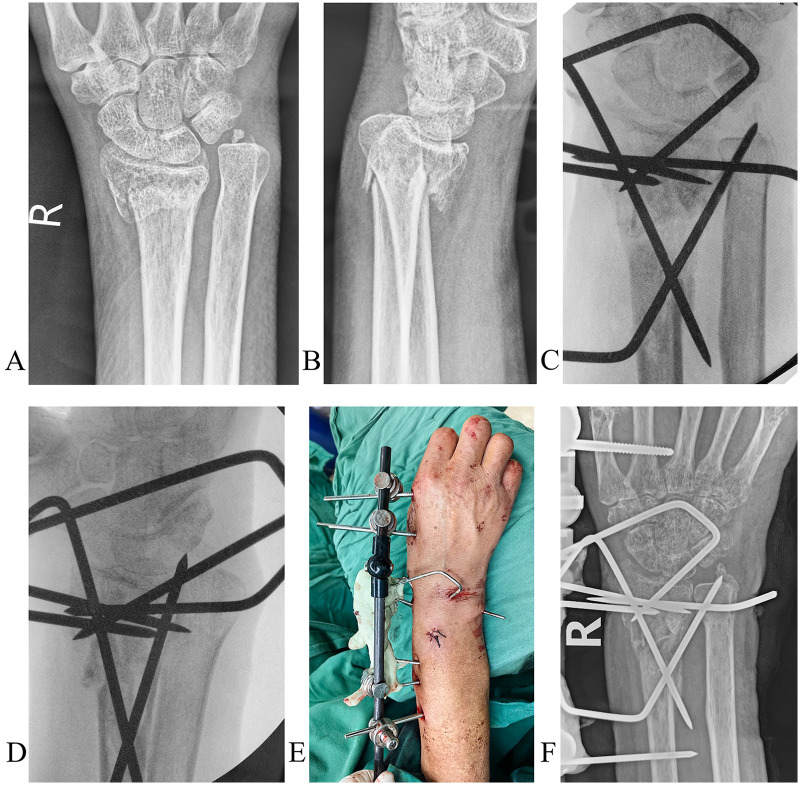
A 58-year-old female patient suffered depressed distal radius malunion (initial type A2) 3 months after fracture. (A) A PA X-ray. (B) A lateral X-ray. (C) A PA X-ray taken immediately after surgery. (D) An oblique X-ray. (E) A photograph showing the dorsal aspect of the forearm. (F) A PA X-ray taken 2 months after correction.
